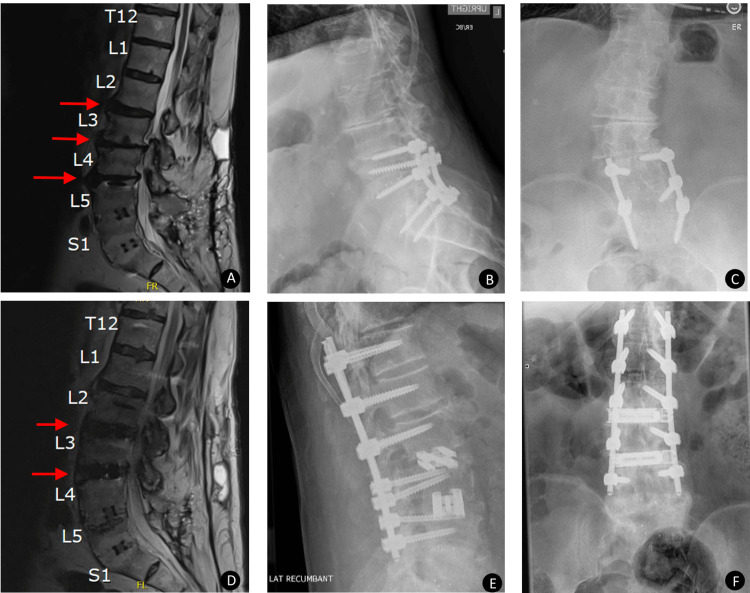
Case 1 postoperative radiographic evaluation of dual expandable spacer and screw placement A 73-year-old male presented with neurogenic claudication and low back pain. Preoperative sagittal magnetic resonance imaging (MRI) (A) demonstrating L3-L5 intervertebral disc desiccation and height loss (red arrows). Preoperative lateral (B) and anteroposterior (AP) (C) x-ray images showing left-sided predominant endplate osteophytosis, sclerosis, and disc height narrowing of L2-L4 with dextroscoliosis of the lower lumbar spine. Instrumentation from prior surgery is also visible. Postoperative sagittal MRI (D) showing postsurgical changes related to posterior spinal fixation hardware from T12-L4 with interbody spacers at L2-4 (red arrows). There is a minimally displaced L5 vertebral body fracture that abuts the previous hardware tracts. Postoperative lateral and AP x-ray images show intact spacers and maintained disc space height without subsidence (E, F).
